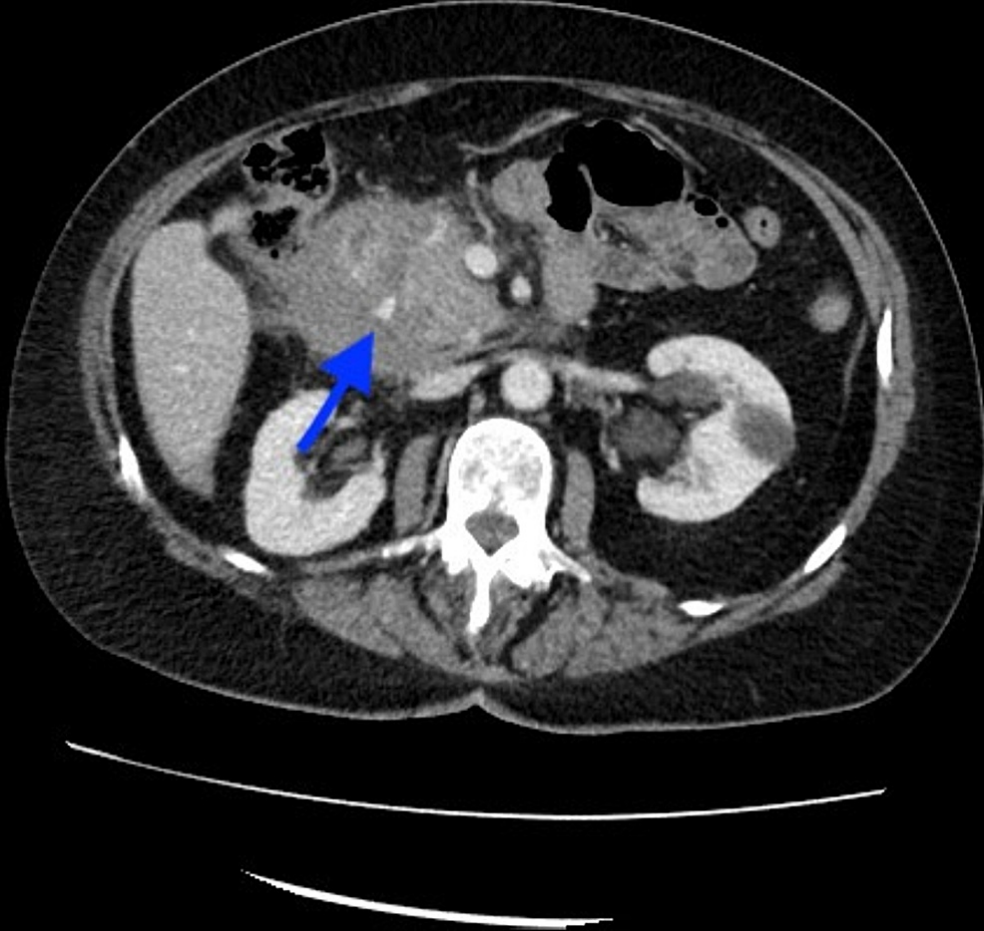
Axial CT demonstrates a large hematoma in the periduodenal region with a focus on active extravasation (blue arrow).
